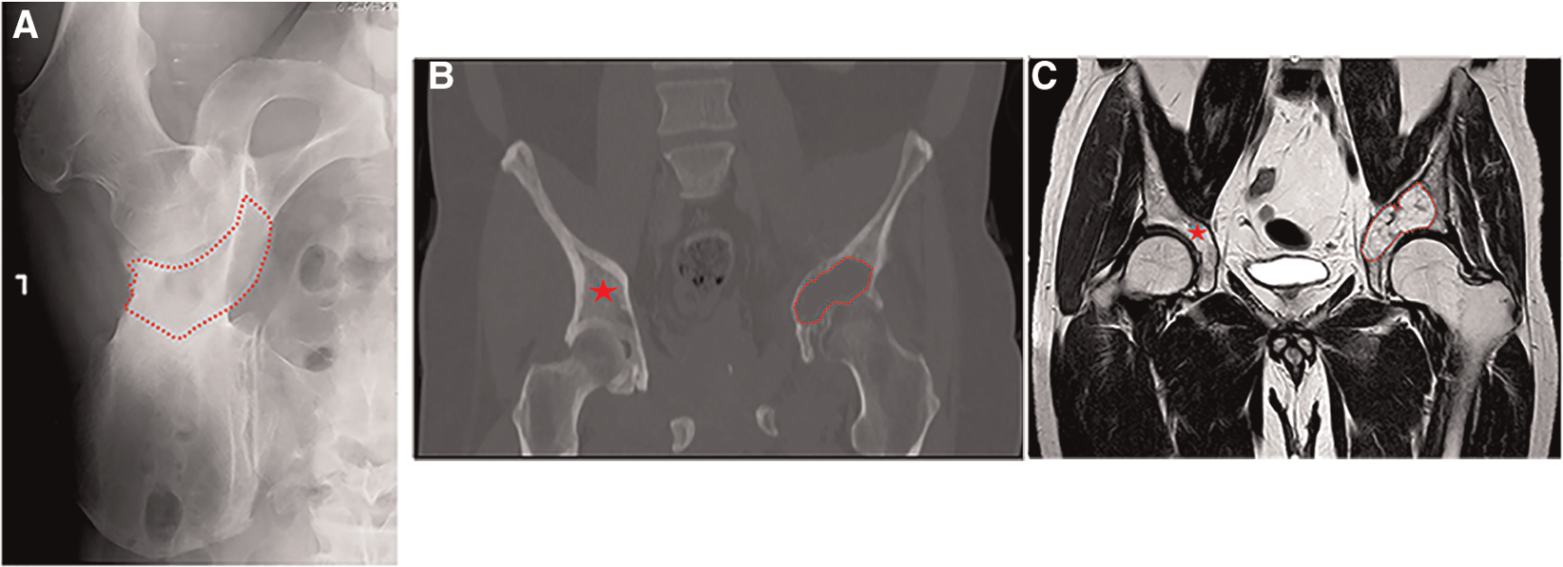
(A) plain anterior-posterior (AP) radiograph of a left hemipelvis, showing high-grade chondrosarcoma of the acetabulum with the destruction of the medial acetabular wall. The red dotted line delimits the radiolucent area created by the bone tumor. (B,C) A coronal computed tomography (CT) and T1 sequence of magnetic resonance imaging (MRI) retrospecivaly, of high-grade chondrosarcoma of the acetabulum with destruction of the medial acetabular wall (red dotted line) and the contralateral healthy bone area (red asterisk).
